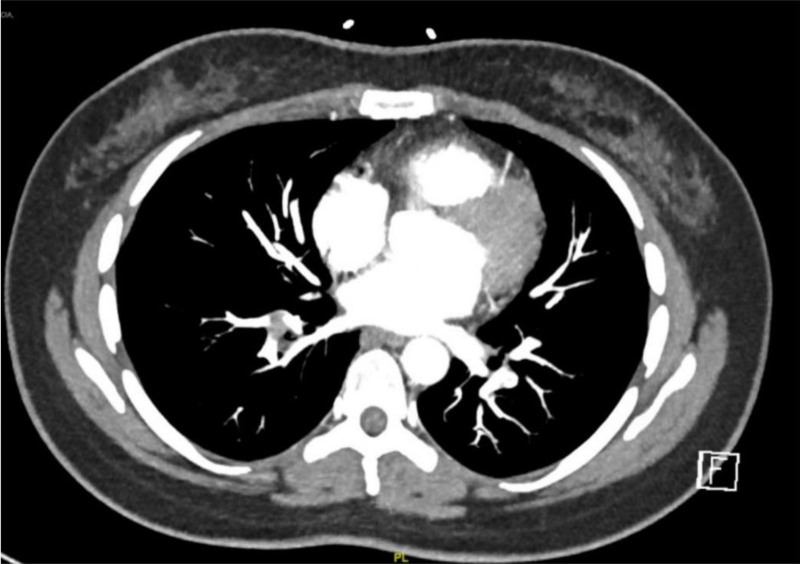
Chest CTA showing bilateral PE. CTA = computed tomography angiography, PE = pulmonary embolism.
